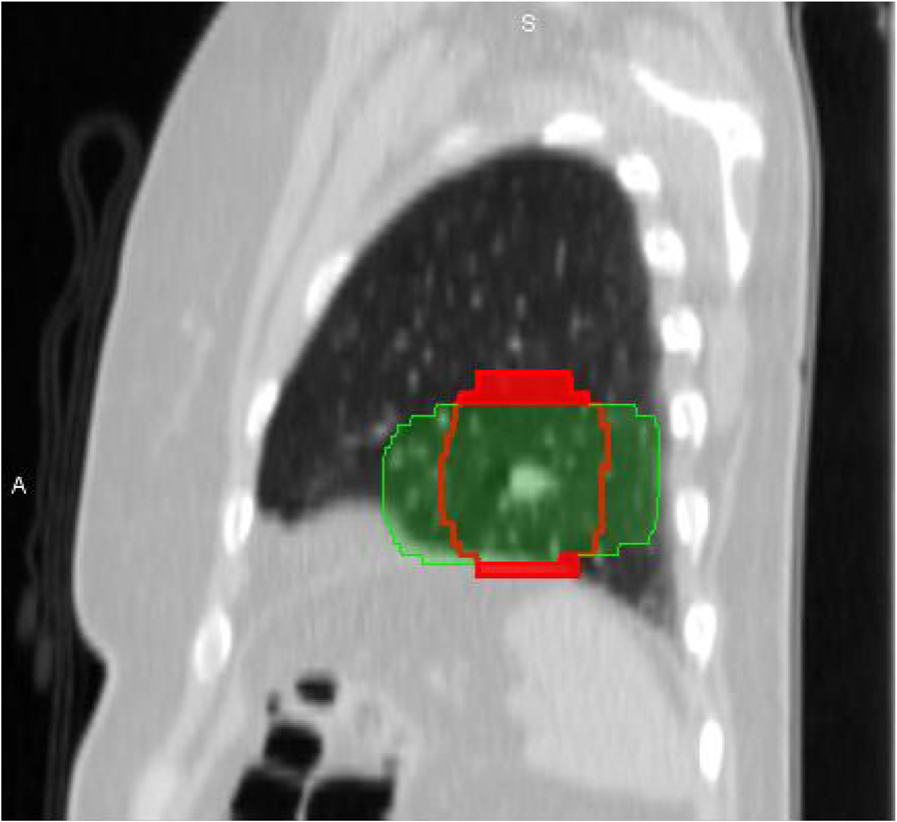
A CT scan of a patient with a small lower lobe tumour. The 4D-PTV is shown in red and the green area show the 95% isodose line. The solid red area is the part of the 4D-PTV that would not receive the prescribed dose and constitutes a Type 3 geographic miss.
